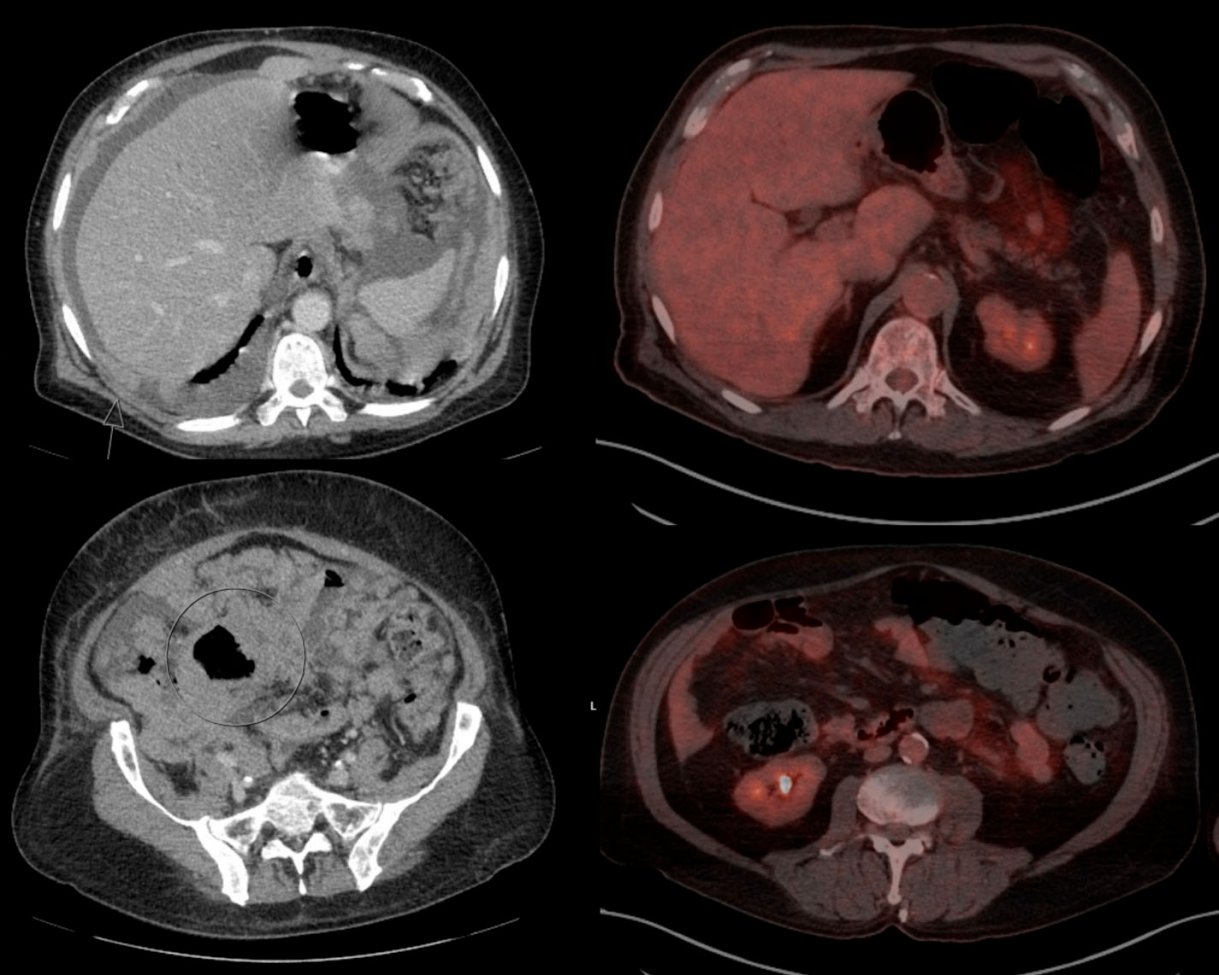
(Left) Initial CT chest, abdomen, and pelvis with contrast demonstrating nodular lymphadenopathy throughout the pleural and peritoneal cavity, including the diaphragm and circumferential distal colonic involvement. Measurable lymphadenopathy notable at the porta hepatis measuring approximately 40 × 29 mm and at the cardiophrenic interface measuring approximately 4.6 × 2.1 cm. (Right) Follow up positron emission tomography and computed tomography (PET/CT) demonstrating complete remission after 2 cycles of DA‐R‐EPOCH.
